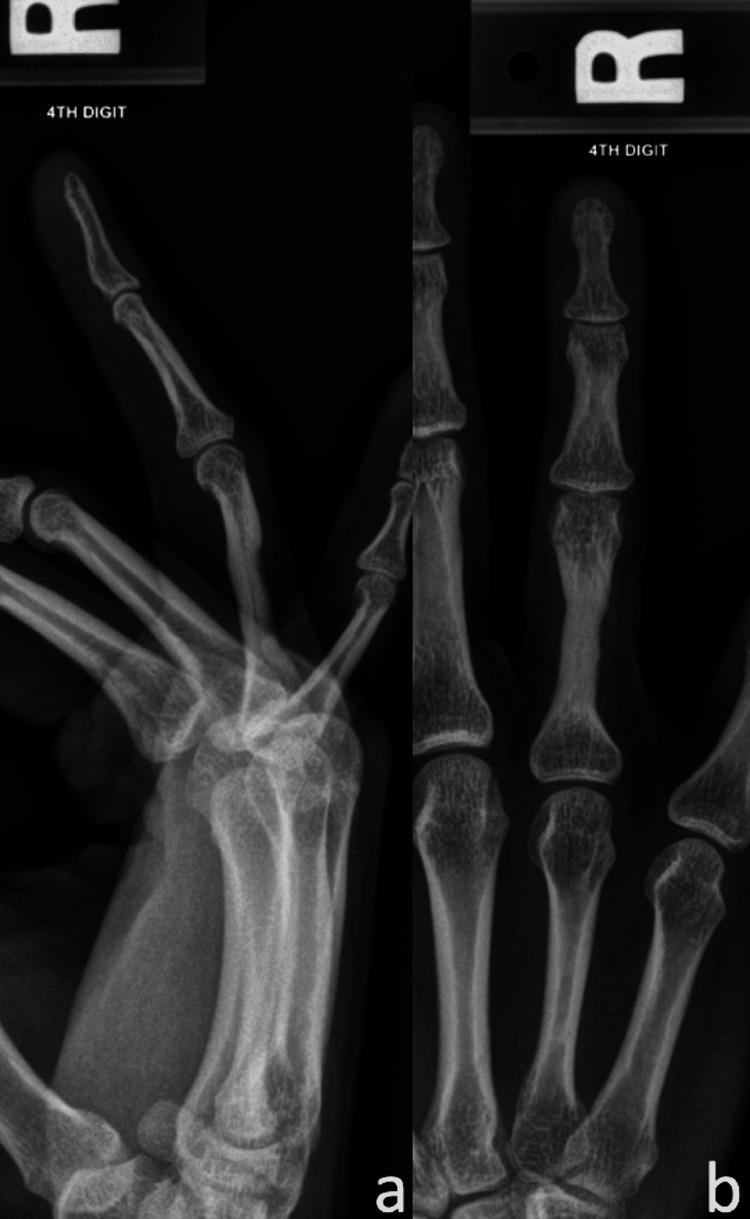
Radiographs at 10 months postoperatively demonstrating remodeling of the proximal phalanx with improved cortical density.
